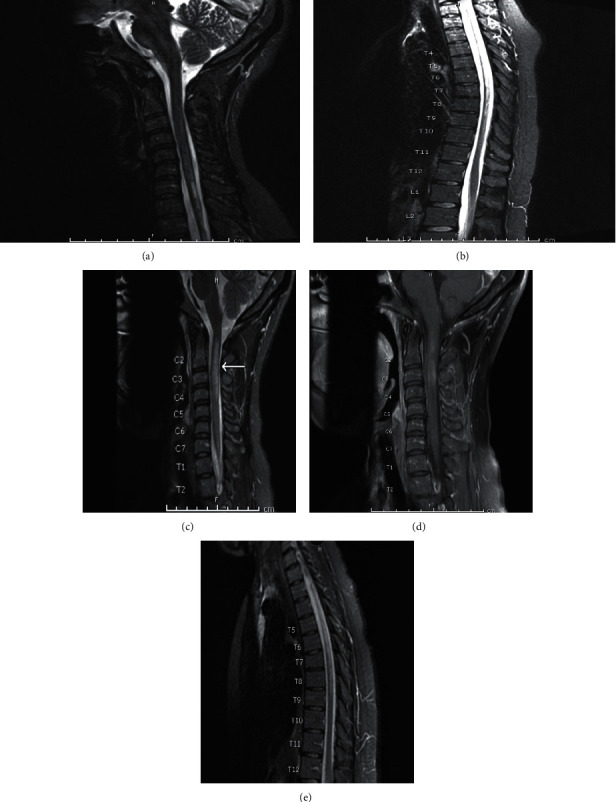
T2WI of cervical and thoracic spine of a 31-year-old African American woman with NMOSD and SLE. In 2018, cervical (a) and thoracic spine (b) demonstrated a C6 hyperintense lesion extending to T8 (a, b), which was unchanged from a prior spine MRI of the patient (not shown). In 2019, there was a new C2–C4 longitudinal lesion noted on the C spine on T2WI (c) with associated patchy postcontrast enhancement on T1WI (d) and an ongoing hyperintense lesion in the T spine extending to T8 (e).
